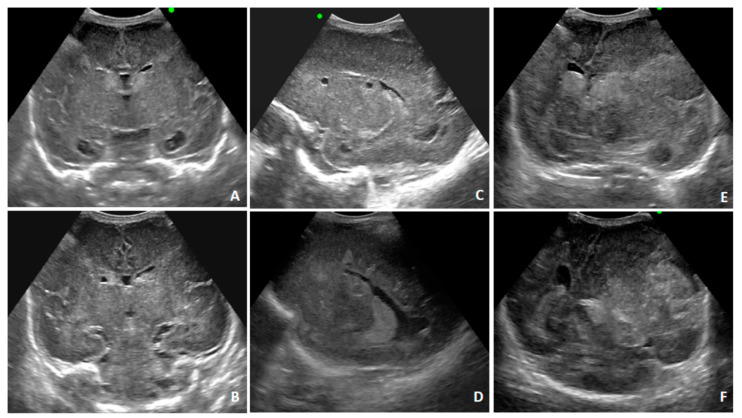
Transfontanellar ultrasound findings for Case 2 at birth. Second day after birth; (A–D): multiple subependymal cysts; temporal horn cysts. Bilateral microhemorrhagic alterations in the thalamic–caudate groove (germinal matrix). Diffuse white matter hyperechogenicity. Two days later; (E,F): bulky hemorrhagic lesions in left cerebral hemisphere with an extraparenchymal component appearing, causing an important mass effect and contralateral midline shift.
